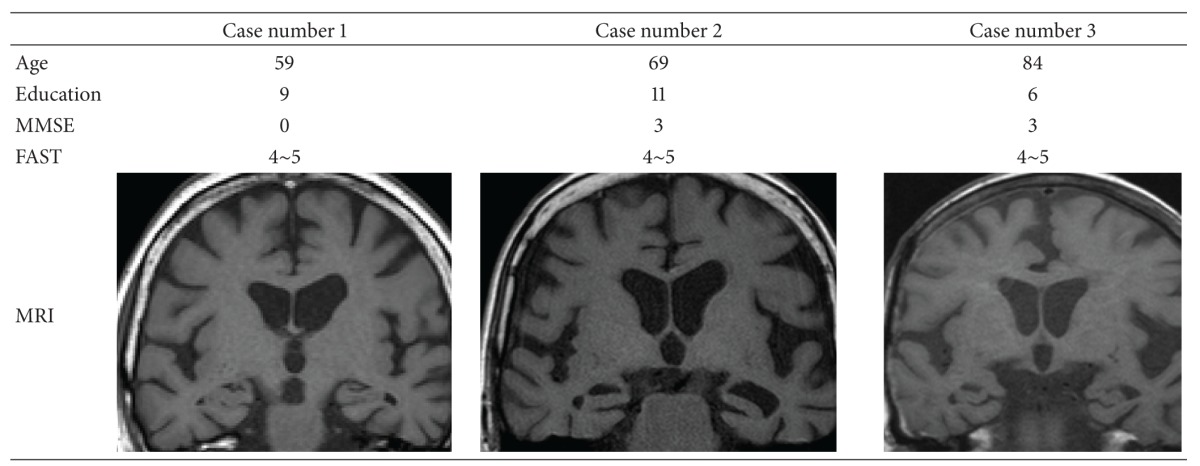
SD: semantic dementia; MMSE: mini mental state exam; FAST: functional assessment staging of Alzheimer's disease; MRI: magnetic resonance imaging.
